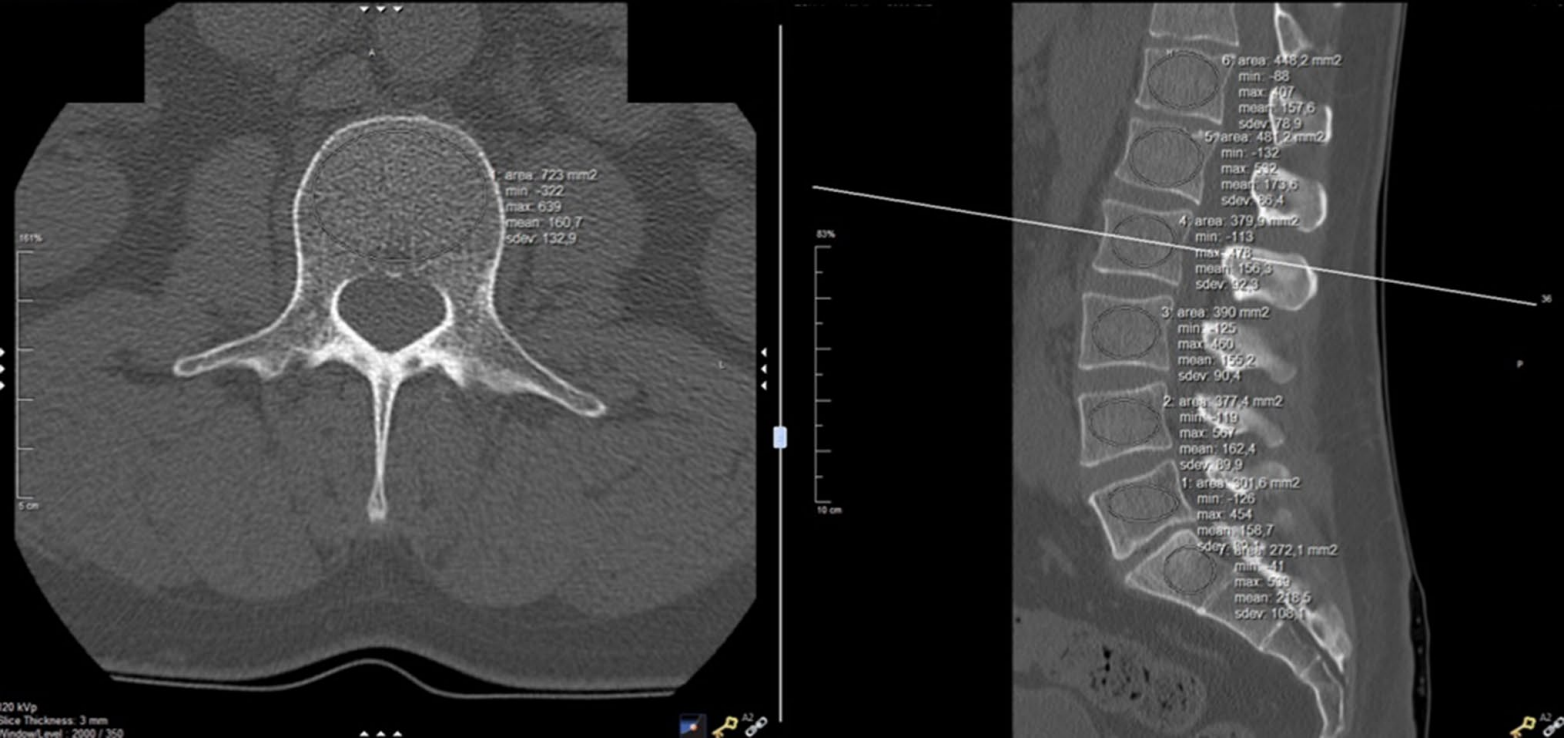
Computed tomography of the spine with axial (left), sagittal (right) measurement of the Hounsfield units using an elliptical measurement field in the center of the vertebral bodies
